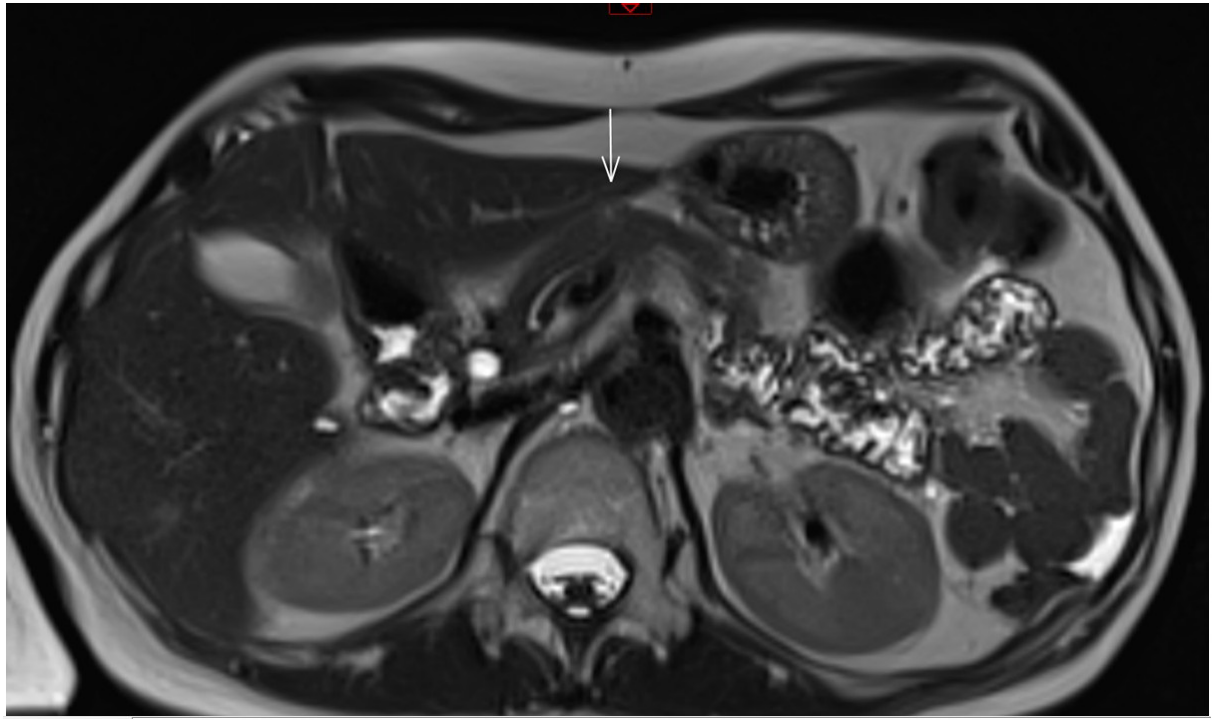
Initial presentation of pancreatic metastasis. Focal subcentimeter lesion on the ventral aspect of the pancreatic body, showing no diffusion restriction or post-contrast enhancement on axial T1-weighted images, and appearing isointense on axial T2-weighted images.
